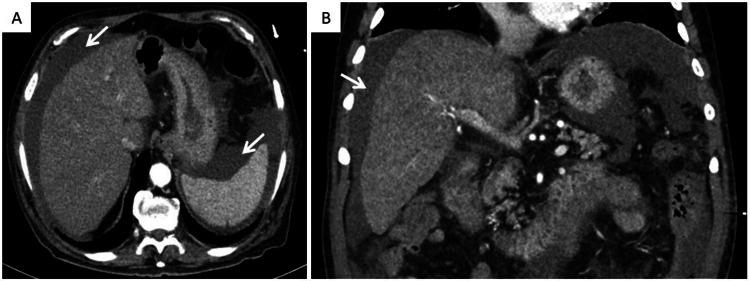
Abdominal computed tomography angiography excluding signs of chronic liver disease, Budd-Chiari syndrome, or malignant infiltration but revealing moderate ascites (white arrows). A) Axial plane; B) Coronal plane
